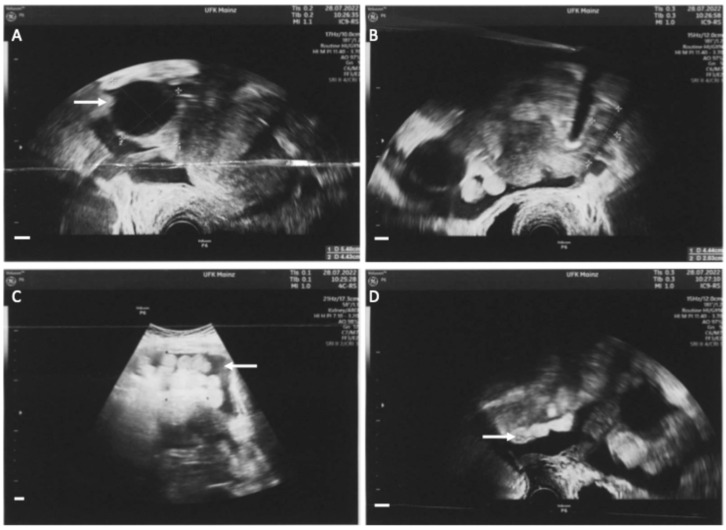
Sonographic assessment at first presentation in our clinic. (A): Enlarged right ovary with an unilocular tumor of 5.4 × 4.4 cm with low echogenicity and a papillary structure (white arrow). (B): Left ovary without pathologic findings. (C): Ascites (white arrow). (D): Peritoneal carcinosis (white arrow). The combination of these findings (ovarian tumor, ascites and peritoneal carcinosis) are potentially indicative of ovarian cancer. All white bars in the bottom left-hand corner indicate 1 cm.
